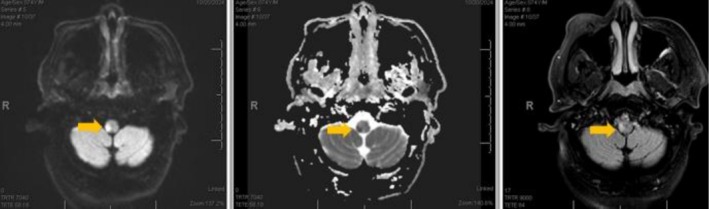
MRI brain without contrast: DWI (left), ADC (middle), flair (right).
